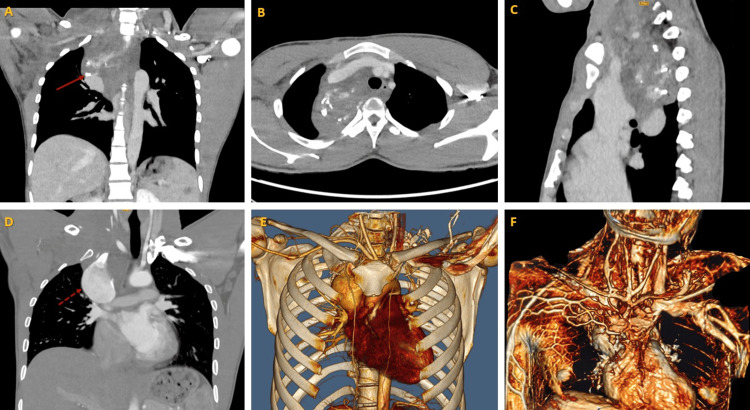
Computed tomography (CT) angiogram of mediastinal mass CT aortic angiogram showing the mediastinal mass that extends above the clavicle in coronal (A), axial (B), and sagittal (C) views; the presence of superior vena cava (SVC) dilation in the coronal CT view (D) and 3D reconstruction view (E); and the presence of superficial dilated chest veins (F). Phleboliths are seen (red solid arrow, A). SVC aneurysmal dilatation is seen (red dotted arrow, D).
